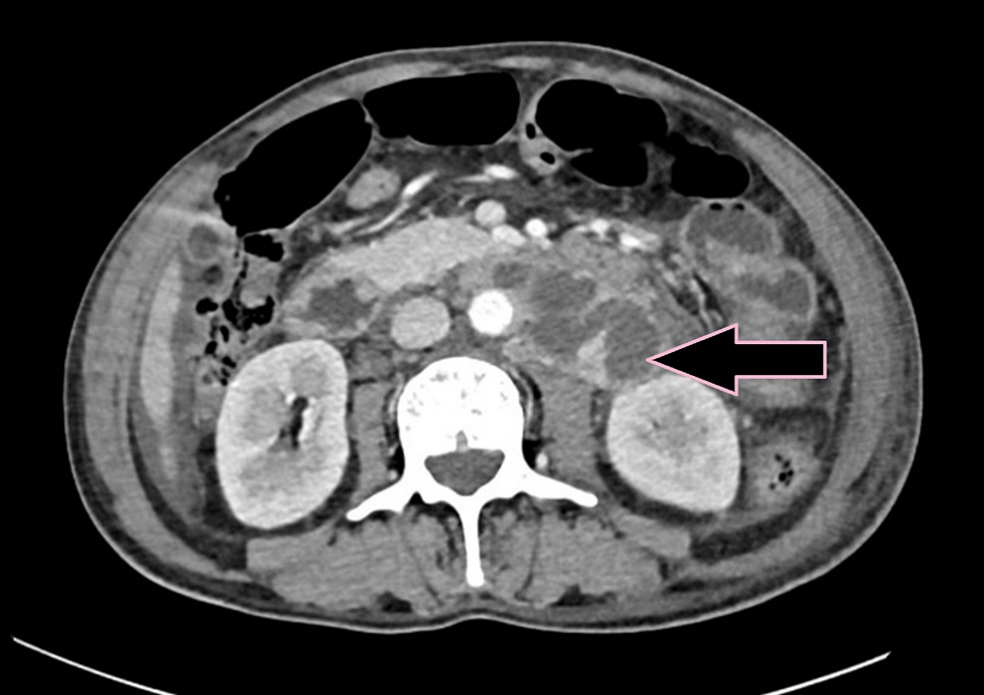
MDCT: contrast-enhanced CT scan shows a lymph node with peripheral enhancement and a low-attenuation center (case 3). MDCT: multidetector computed tomography
